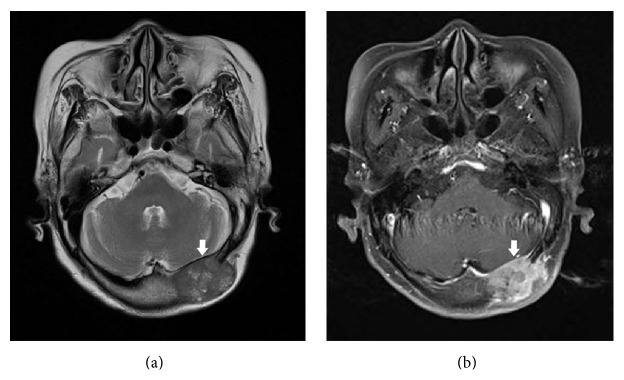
(a) T2W and FLAIR axial image. (b) T1W axial image with Gd-DTPA. Relatively well-defined lobulating, enhancing skull mass in left occiput (3.5 × 2.5 cm), with destruction of bone and intracranial extension compressing adjacent sinus, but no definite brain invasion.
